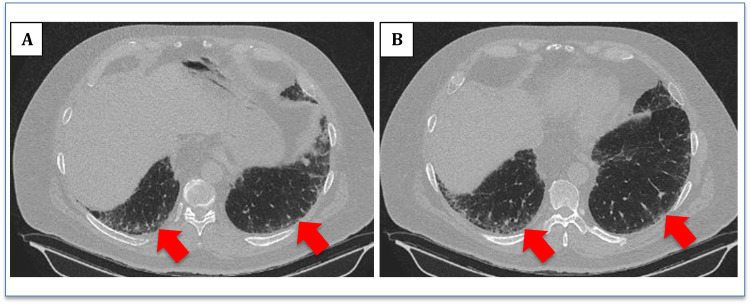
(A, B) CT scan of the chest (lung window) showing mild subpleural fibrosis (red arrows)
